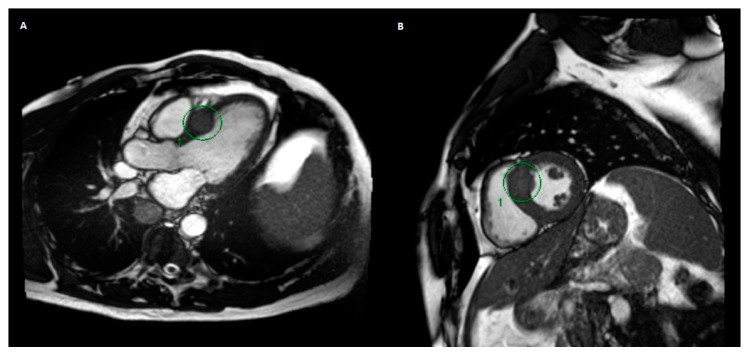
MRI of the thorax and upper abdomen done three months after radiation treatment, showing stability of the metastatic myocardial lesion (circled). No gadolinium contrast was used for this scan, as the patient had an acute kidney injury at the time. Representative axial (A) and sagittal (B) images are shown on the left and right, respectively.
